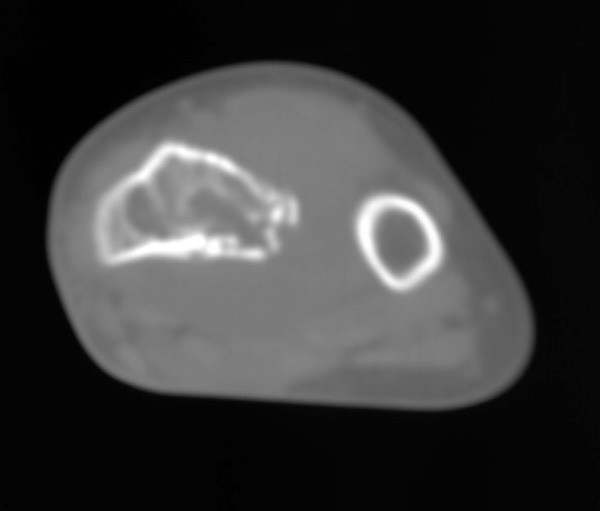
Initial evaluation. CT: lytic lesion with partial cortical destruction.
